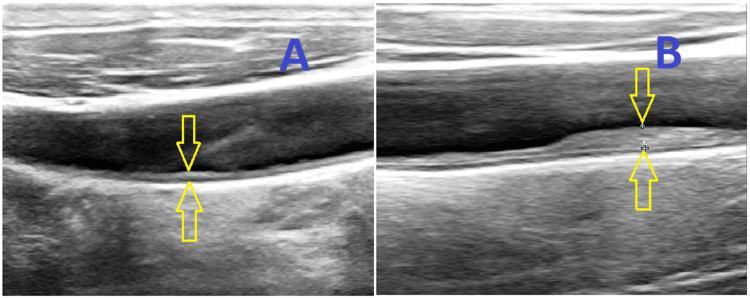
Duplex ultrasonography (B-mode image) shows the distances between the intimal-luminal (upper arrow) and the medial-adventitial interfaces (lower arrow). CIMT (A) and carotid plaque (B). CIMT: carotid intima-media thickness.
